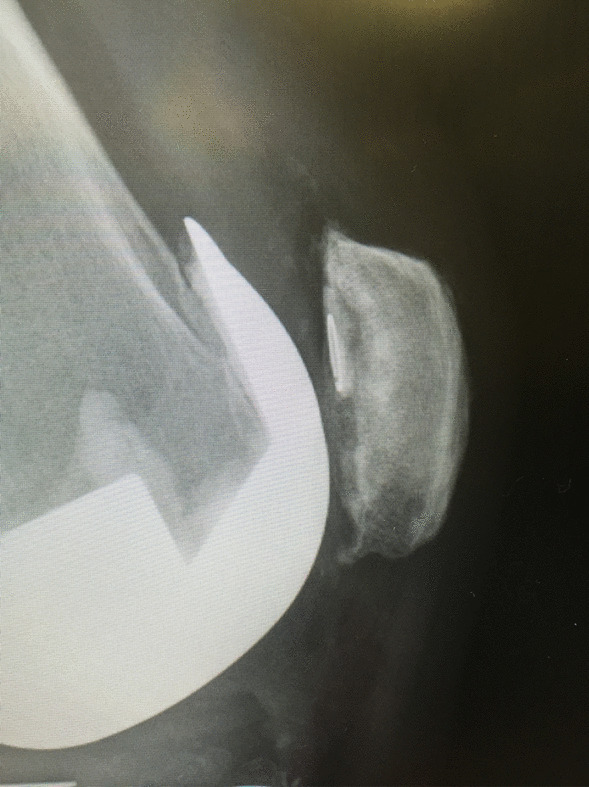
Patella loosening on preoperative radiographs of a 55-year-old male patient undergoing patella revision
